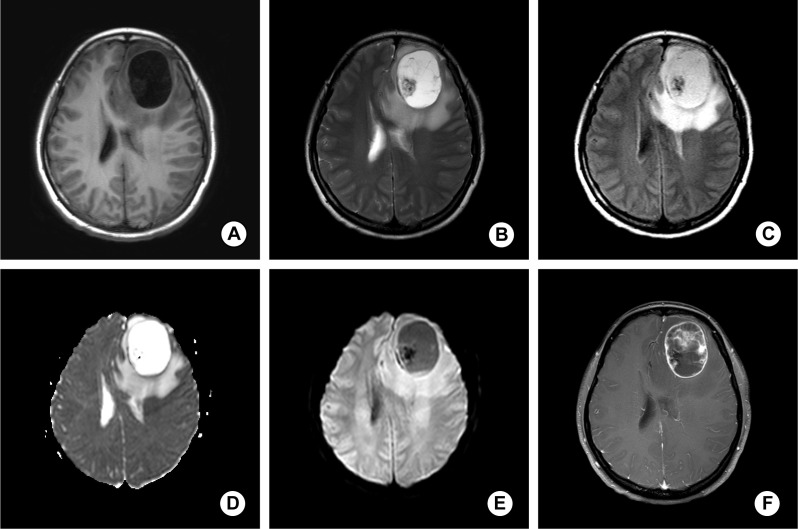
(A) MRI showed the tumor located in the frontal lobe was a well-circumscribed mass, and the lesion was predominantly hypointense with an isointense solid nodule on T1-weighted images. (B) The tumor appeared predominantly hyperintense with the solid component showing iso- to hypointense signals on T2-weighted images. (C) The tumor was hyperintense on FLAIR sequences. (D) The tumor showed no diffusion restriction on DWI. (E, F) Post-contrast T1-weighted fat-suppressed images showed peripheral rim enhancement and patchy enhancement of solid components.
